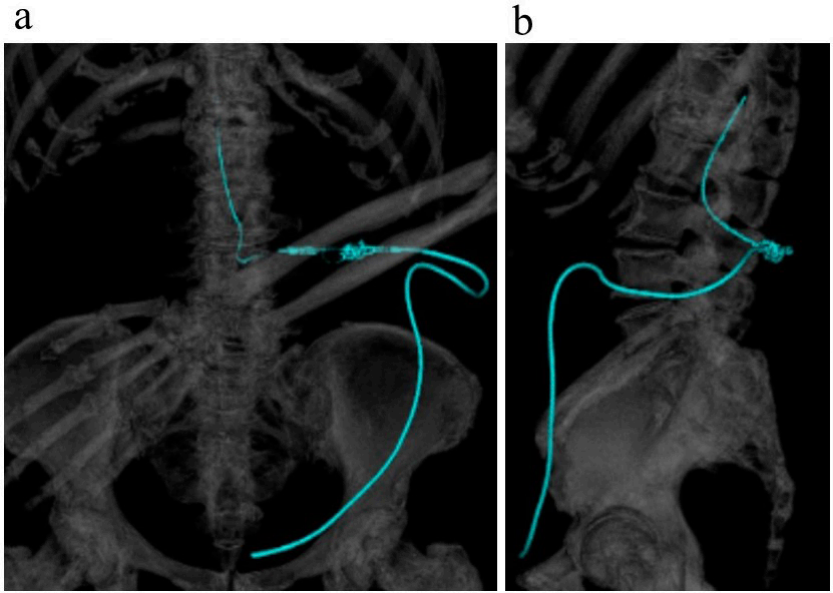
Postoperative three-dimensional computed tomography showed the programmable valve and catheter’s correct placement.
